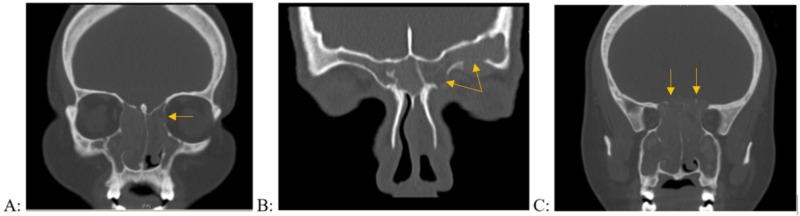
Application of the bone erosion grading system on computed tomography images of the paranasal sinuses in patients with allergic fungal rhinosinusitis (A) Grade 1 erosion of the left lamina papyracea, (B) Grade 2 erosion of the left frontal floor, (C) Grade 3 erosion of the posterior ethmoid roof on the left and right sides
